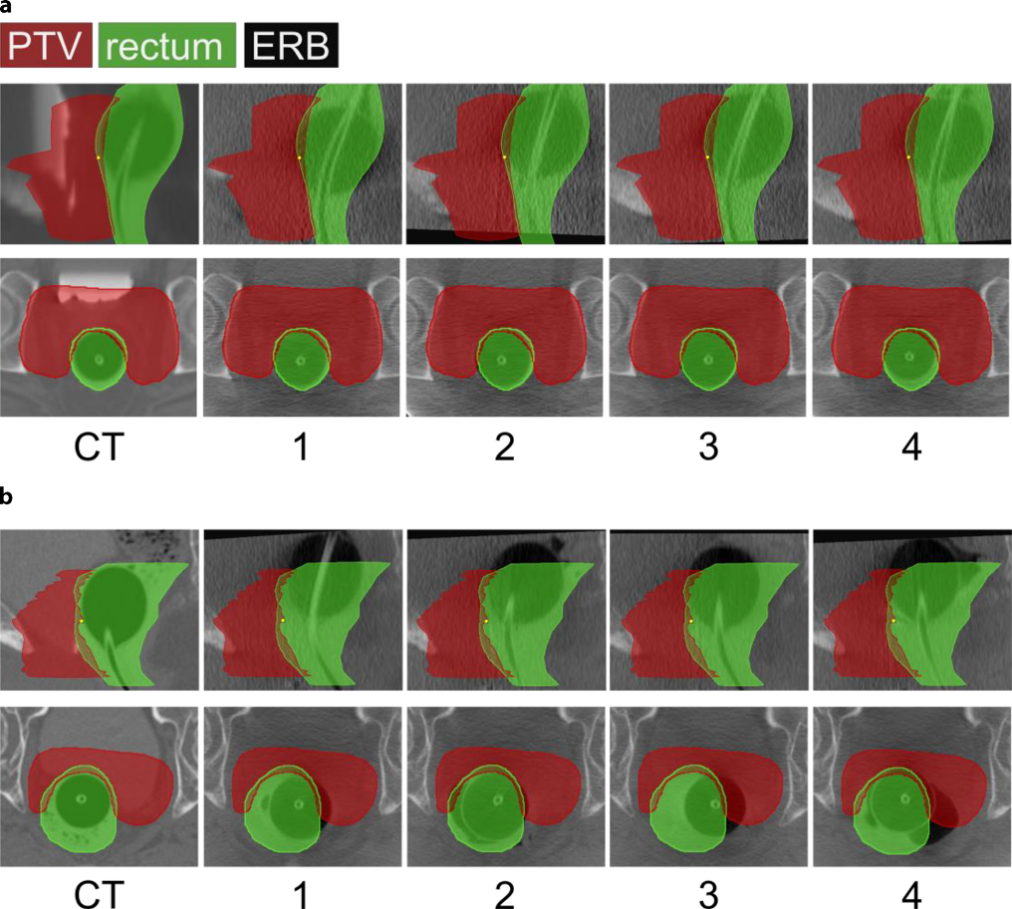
Example of patient positioning for the first 4 treatment days. The planning CT (computed tomography) on the far left represents the reference for the following CBCTs rigid registration. In a, a patient with a low setup error and in b a patient corresponding to larger positioning variation are displayed. For b offline ART was applied. *PTV* planning target volume, *ERB* endorectal balloon
